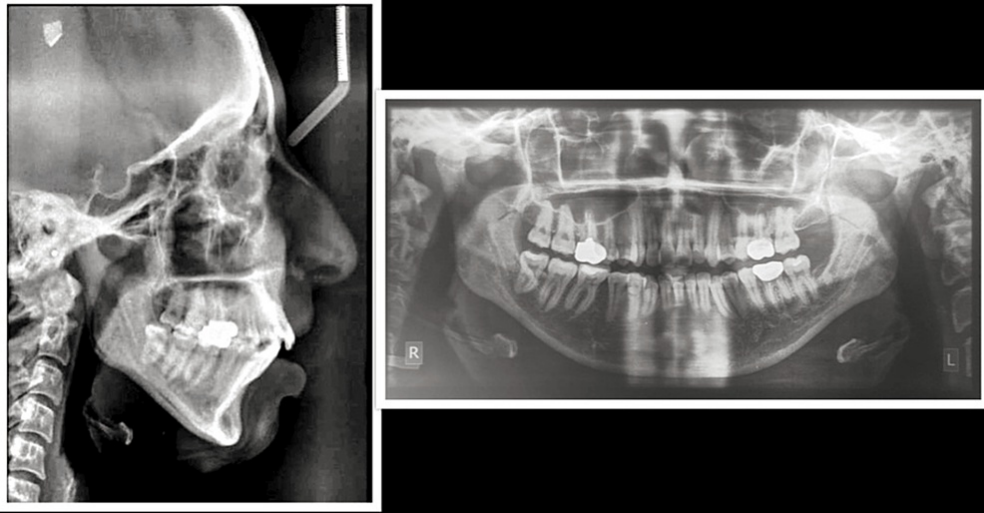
Pretreatment radiographs U1-SN: 114.666 degree; L1-Apog: 8.444 mm; L1-NB: 35.666 degree; L1-NB: 11.777 mm
